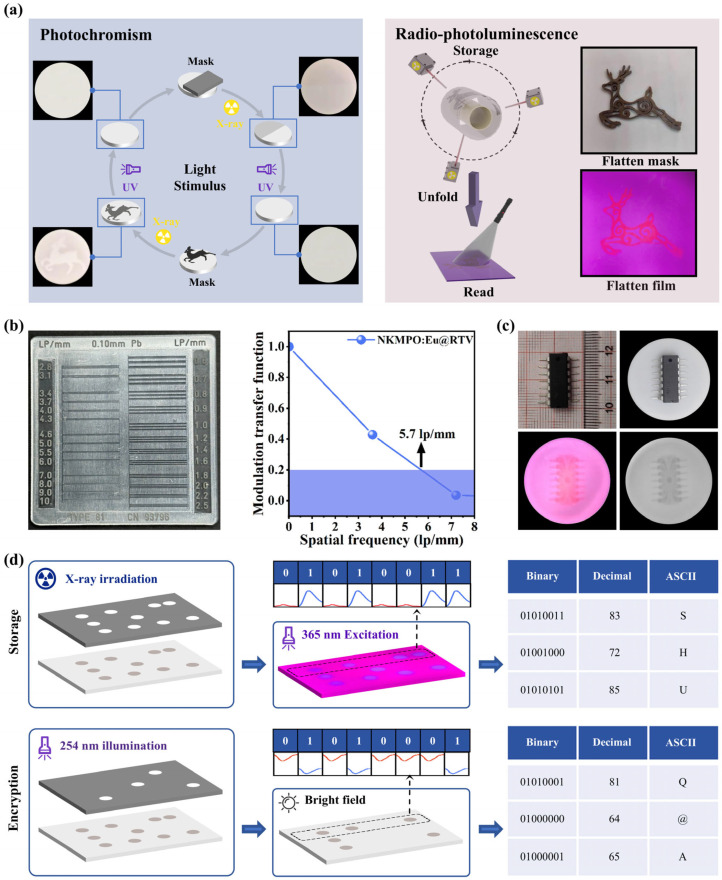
(a) Photochromism and radio-photoluminescence images of the NKMPO:Eu@RTV film. (b) Standard line pair card (left) and gray value modulation (right). (c) Detailed X-ray images of CMOS captured with a color camera and a black-and-white camera. (d) Information encryption application based on the mutual modulation of photochromism and radio-photoluminescence.
